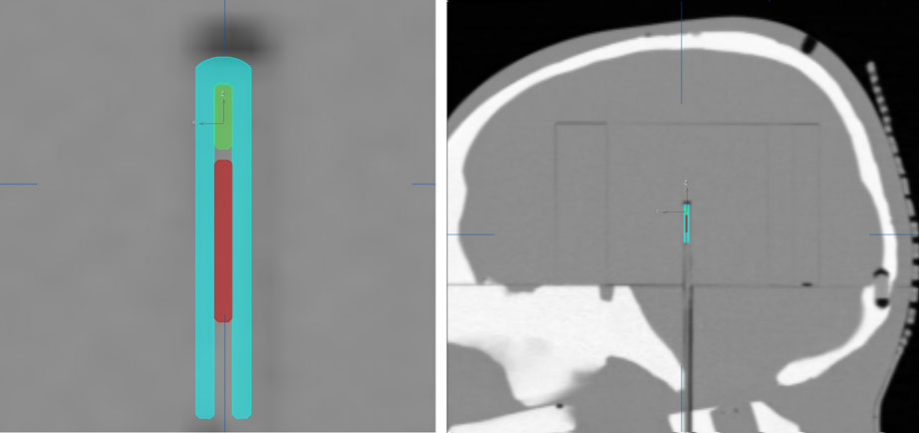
Model of W1 detector within the BRUNO CT dataset. The ABS shell is shown in teal, the fluorescent‐doped polystyrene scintillating fiber is shown in green, and the PMMA optical fiber core is shown in red.
